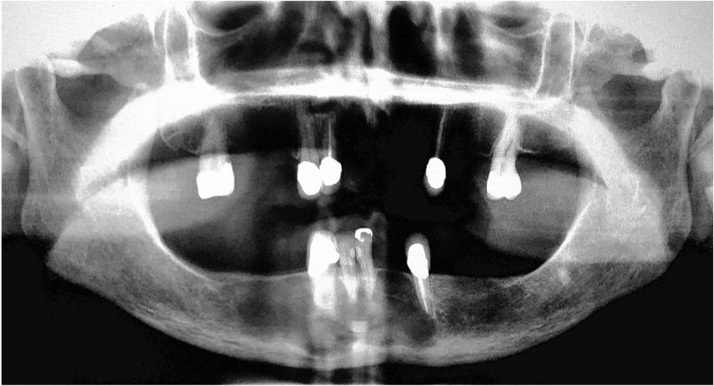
Orthopantomography with evidence of radiolucency in the submental and mental region of mandible bone.
